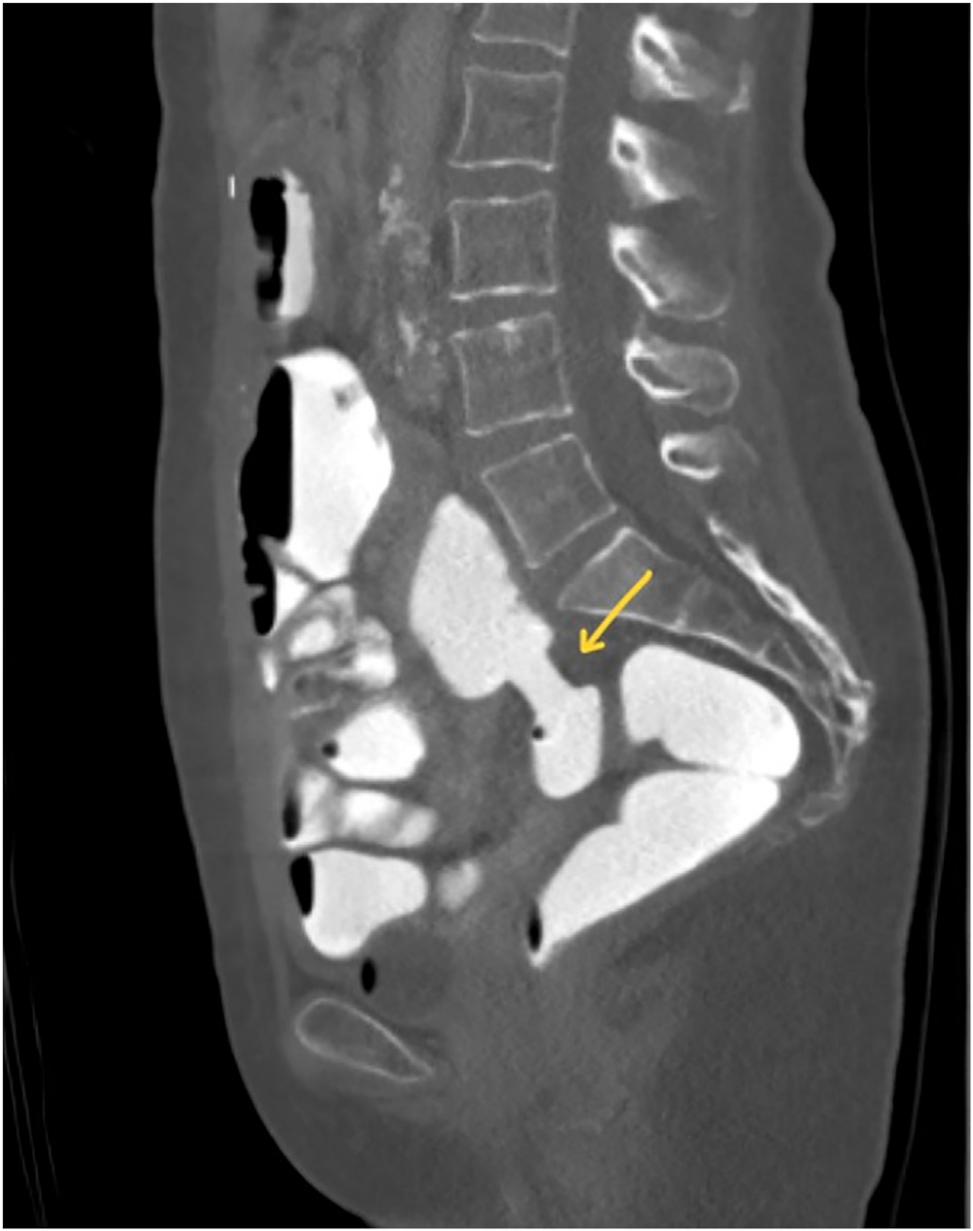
Sagittal CT image of the ileocolostomy (*yellow arrow*) and decompression of the small bowel.
